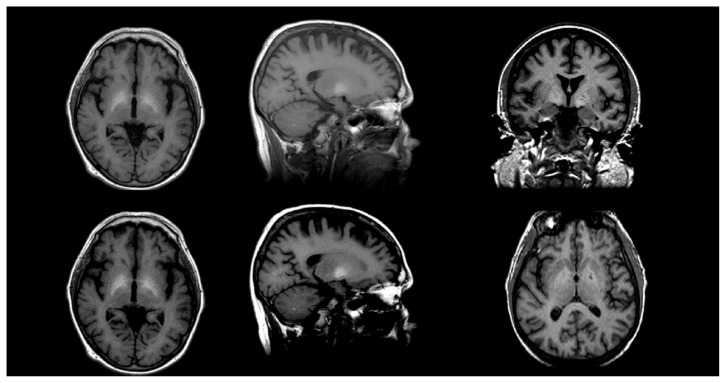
Hepatic encephalopathy (HE). T1-weighted imaging in HE reveals bilateral, symmetrical, high-intensity signals in basal ganglia structures, particularly the globus pallidus and substantia nigra, probably due to manganese deposition and T1 shortening. T2-weighted fluid attenuation inversion recovery (FLAIR) shows hyperintense signals along the corticospinal tract and diffuse hyperintense white matter signal in the cerebral hemispheres.
